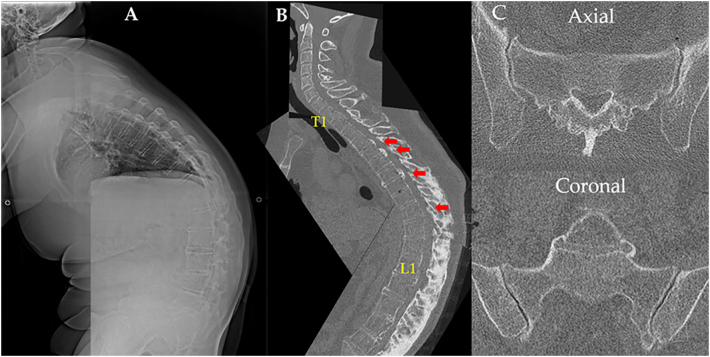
A) Standing sagittal radiograph at the initial examination showing significant kyphosis of the entire thoracolumbar spine and so-called “bamboo spine”. B) Sagittal reconstructed computed tomography at the initial examination showing abnormal hyperostosis in the anterior longitudinal ligaments and massive ossification of the supraspinous ligament. Red arrows indicate segmental type OPLL at T4–8/L1–2 and OYL at T10–11. C) CT scan of the sacroiliac joints showing bilateral narrowing of the joint gap and osteosclerosis. (For interpretation of the references to colour in this figure legend, the reader is referred to the web version of this article.)
